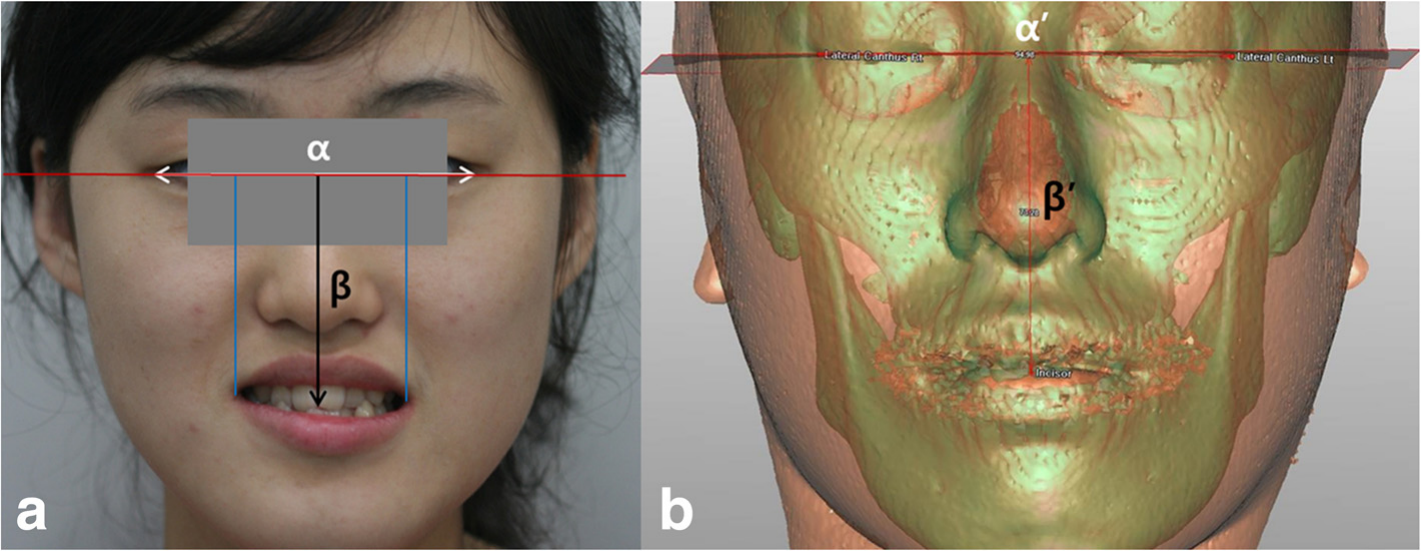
Calibration of a clinical picture of a patient’s smile according to the 3D CT image (e.g., preoperative image). a 2D photograph; b 3D CT image. At first, the lateral intercanthus distance (*white α*) in the smile picture matched that (*white α’*) in the 3D CT image. The vertical ratio of the photograph was modified by adjusting the vertical distance (*black β*) from the lateral intercanthus line to the anterior tooth in the smile picture to match that (*black β’*) in the 3D CT image. The vertical distance of the lips during smiling, which is the shortest distance (*blue line*) from the lateral intercanthus line (*red line*) to the mouth corner in the photograph
